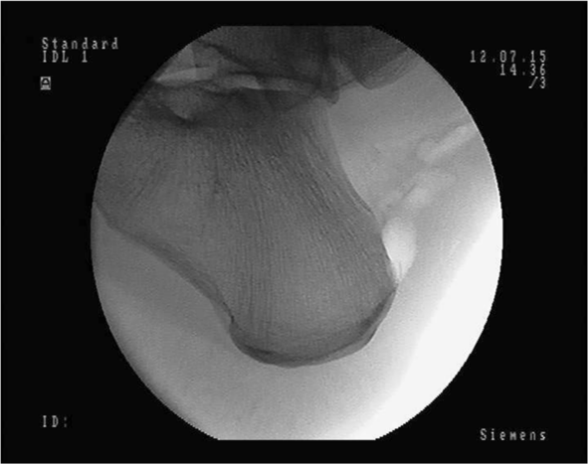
X-ray control of the shape of the calcaneus
